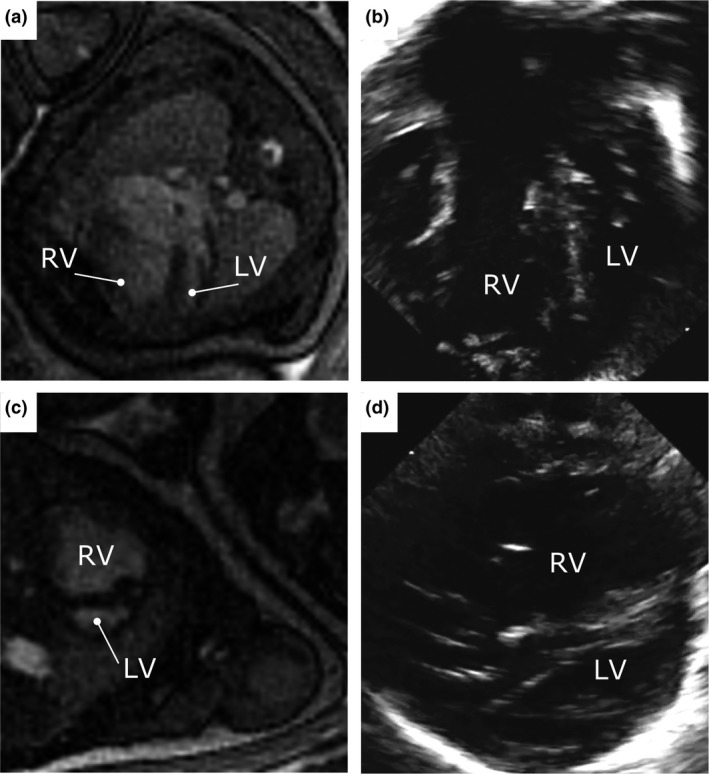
Fetal iGRASP cardiovascular cine magnetic resonance imaging in 4‐chamber (a) and short‐axis (c) views, clearly showing the unbalanced common atrioventricular canal favouring the right ventricle, and ruling out a moderate or large inlet ventricular septal defect or other ventricular septal defect. Images are shown without interpolation. The corresponding postnatal echocardiogram acquired ten days after delivery are shown for comparison in 4‐chamber (b) and short‐axis (d) views, confirming the prenatal diagnosis by iGRASP cardiovascular cine magnetic resonance imaging. LV = left ventricle; RV = right ventricle.
